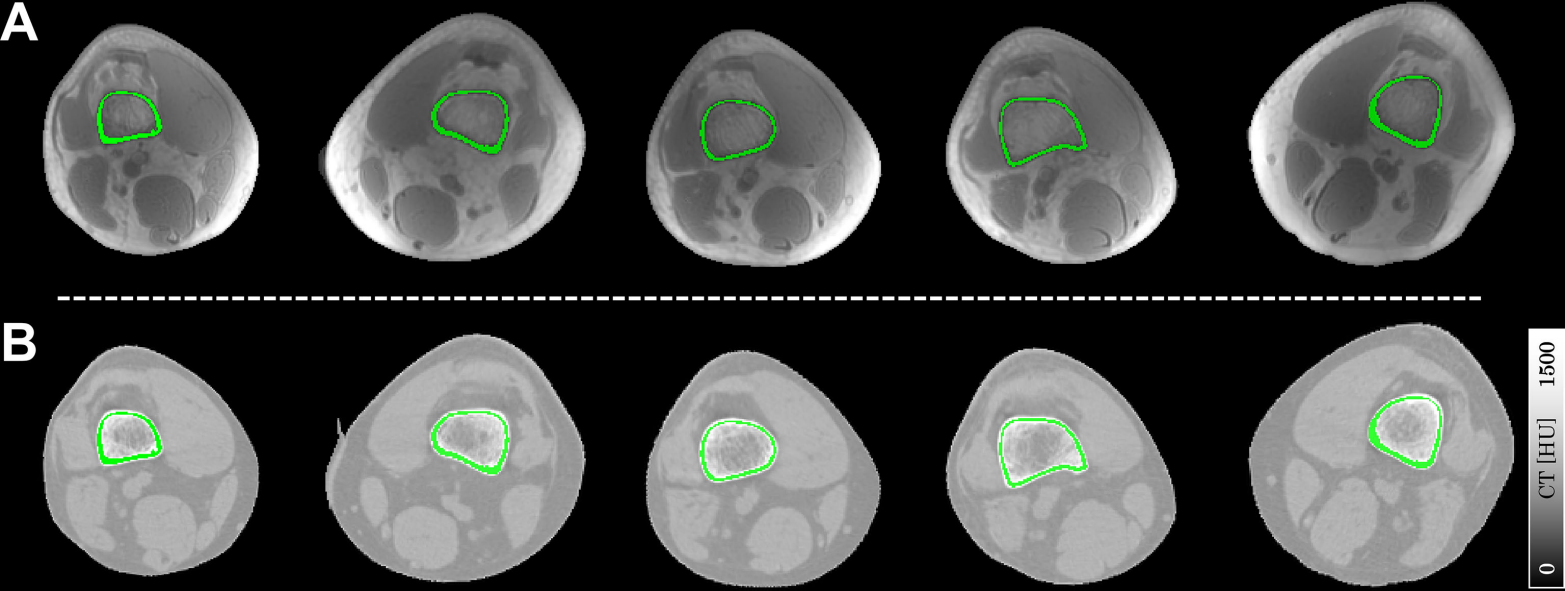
Representative slices of ROIs of cortical bone (in green) overlaid on the UTE magnitude images (A) and registered CT images (B). Each column represents a typical ROI from an individual subject in this study.
